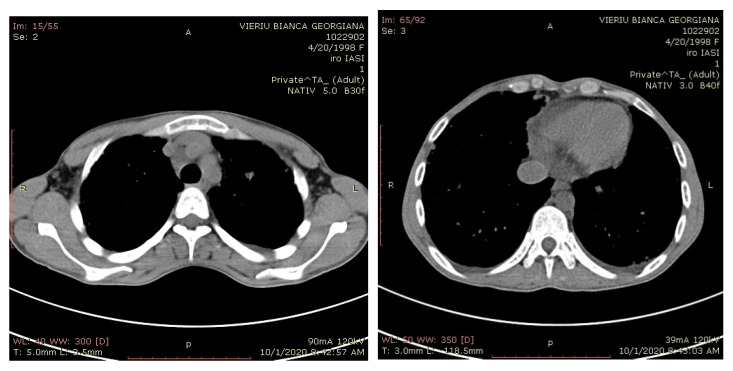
Computer tomography (CT) scans revealed lung metastases.
